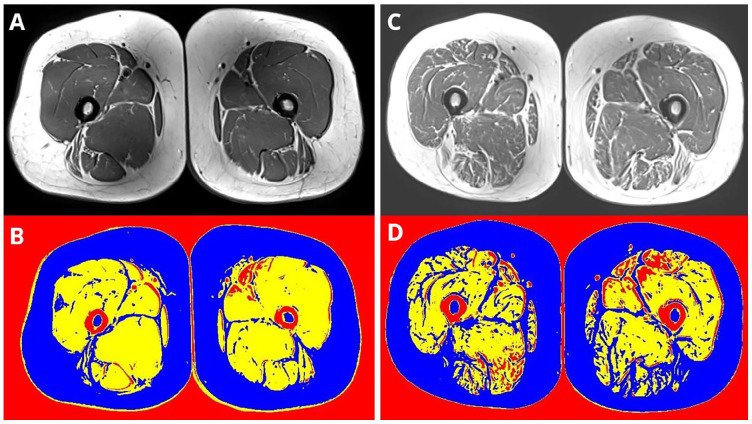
Quantification of muscle, fat, and miscellaneous components of the muscle taken from MRI Figure shows image from original MRI (top row) and then quantification of muscle and fat (bottom row). Figure shows two male study participants with similar thigh area and represents relatively preserved muscle composition (left, images A and B, male, aged 58 years, eGFR 28 ml/min/1.73 m^2^, and poor muscle composition (right, images C and D, male, aged 50 years, eGFR 32 ml/min/1.73 m^2^). Yellow = muscle (contractile), Blue = fat (non-contractile), Red = miscellaneous (connective tissue, fascia) tissue.
